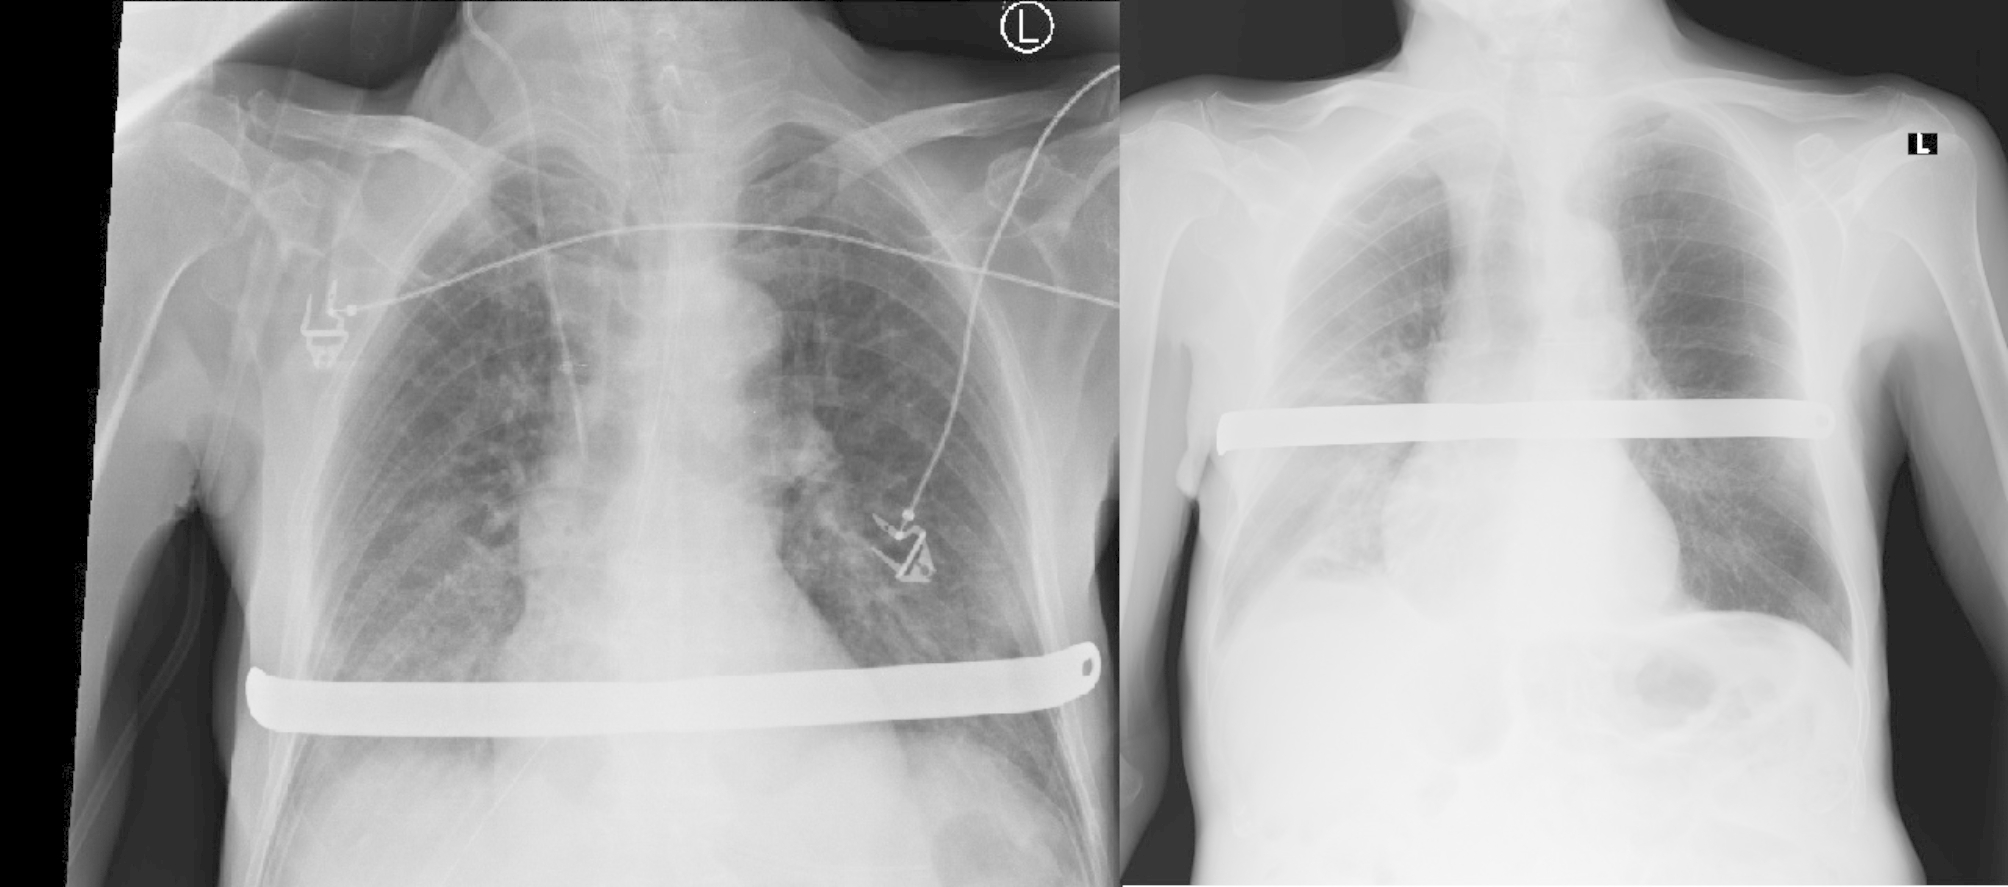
X-ray day after surgery and day of discharge
